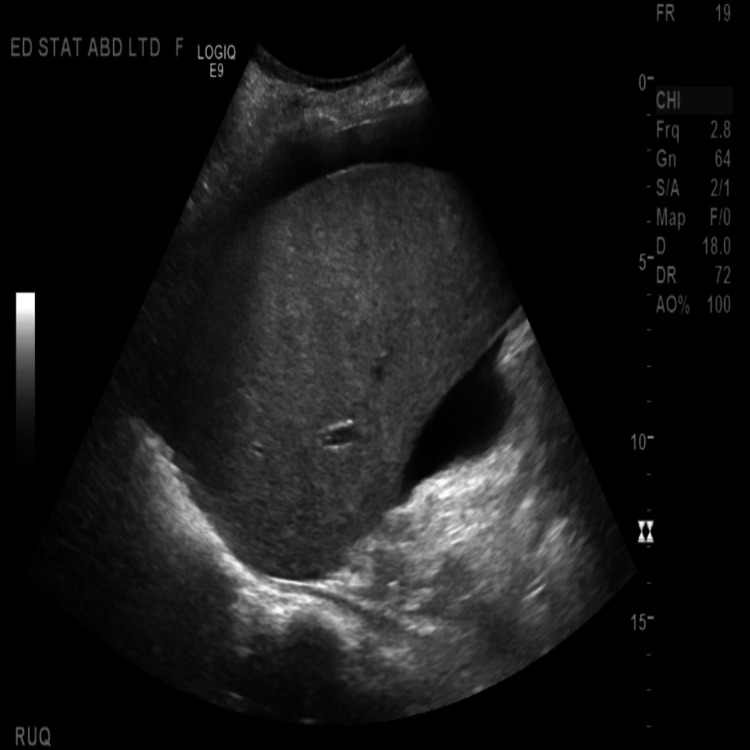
Abdominal US showing a diffusely enlarged liver with minimal nodularity US: ultrasound
